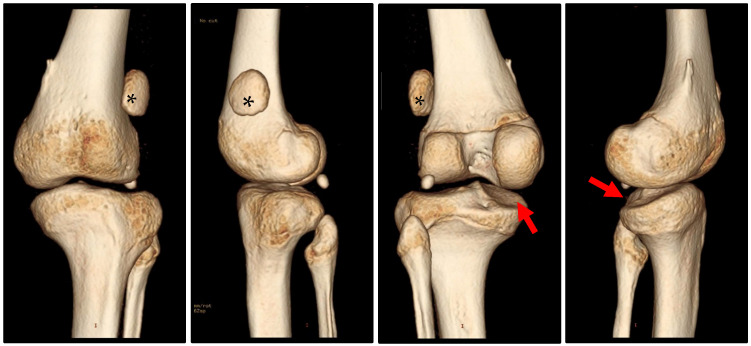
Three-dimensional CT reconstruction showing the laterally dislocated patella (*) and the complex knee deformity with a posterior tibial slope of the medial tibial plateau (arrow).
